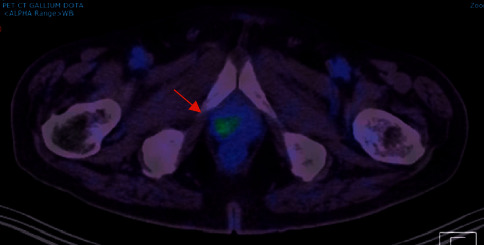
Ga68-DOTANOC PET/CT demonstrating increased DOTANOC avidity seen in the inferior aspect of the right side of the prostate gland (red arrow).
